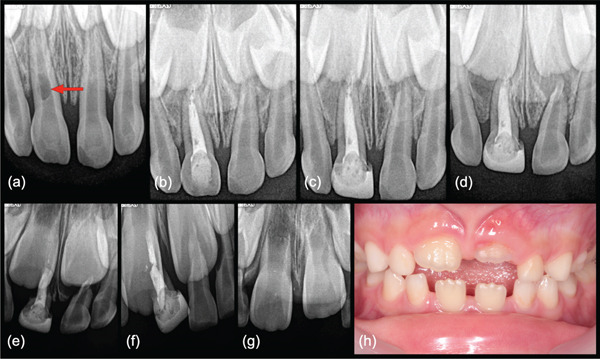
Elective extraction with residual MTA removal (Case 10). (a) Preoperative radiograph of a 3‐year 9‐month‐old symptomatic male patient presenting with history of trauma to the maxillary right primary central incisor (No. D) displaying external root resorption (arrow). (b) Post‐operative radiograph after root canal treatment and MTA obturation. (c) Four‐month post‐operative radiograph after placement of resin strip crown. (d) One‐year, 9‐month radiographic recall. Note arrest of ongoing external resorption. (e) Radiograph showing 2‐year, 10‐month follow‐up. (f) Three‐year, 7‐month radiograph prior to elective extraction. (g) Two‐week follow‐up radiograph after routine extraction and atraumatic curettage combined with water spray irrigation/flushing removal of remaining MTA. (h) Clinical photograph showing normal eruption pattern of the permanent central incisor.
